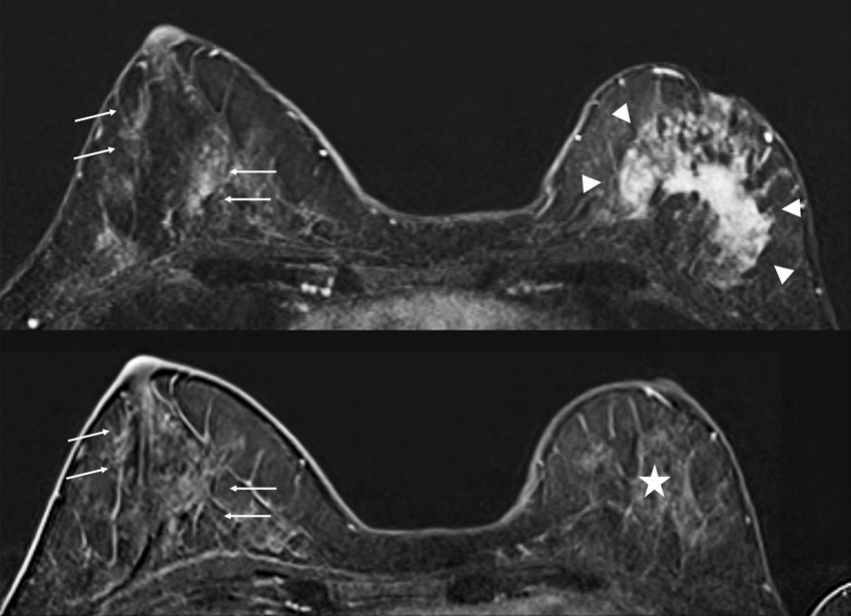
Breast MRI of a 45-year-old premenopausal woman with grade II luminal B HER 2 positive cT2N2 ductal invasive carcinoma relapsing with bone metastases 2 years after surgery, despite having achieved pathological complete response with neoadjuvant chemotherapy. Pretherapeutic MRI (A) showed a 5 cm tumor in the left breast (arrowheads) and moderate BPE, as evaluated in the right breast (arrows). Post-therapeutic MRI (B) showed no residual enhancing tumor in the left breast (star) with persisting BPE (arrows).
